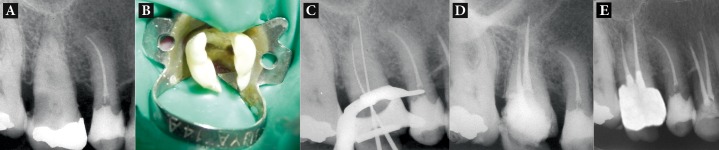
A) Initial radiograph of first maxillary molar with one buccal root; B) Two orifices were detected in the floor of the tooth; C) Working length determination; D) Post-operative radiograph after treatment; E) One-year recall
